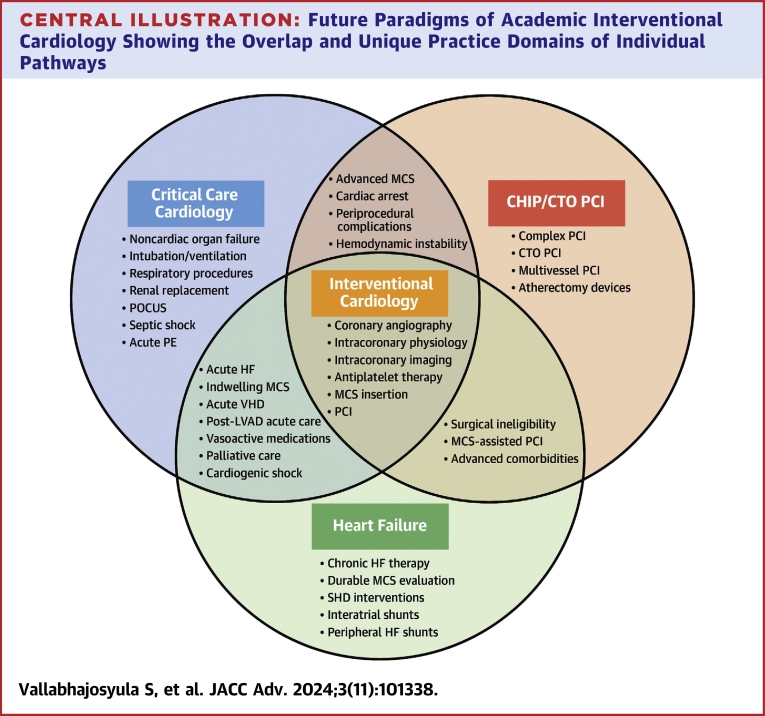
Future Paradigms of Academic Interventional Cardiology Showing the Overlap and Unique Practice Domains of Individual Pathways CTO = chronic total occlusion; HF = heart failure; LVAD = left ventricular assist device; MCS = mechanical circulatory support; POCUS = point-of-care ultrasonography; PE = pulmonary embolism; SHD = structural heart disease; VHD = valvular heart disease; other abbreviation as in Figure 1.
